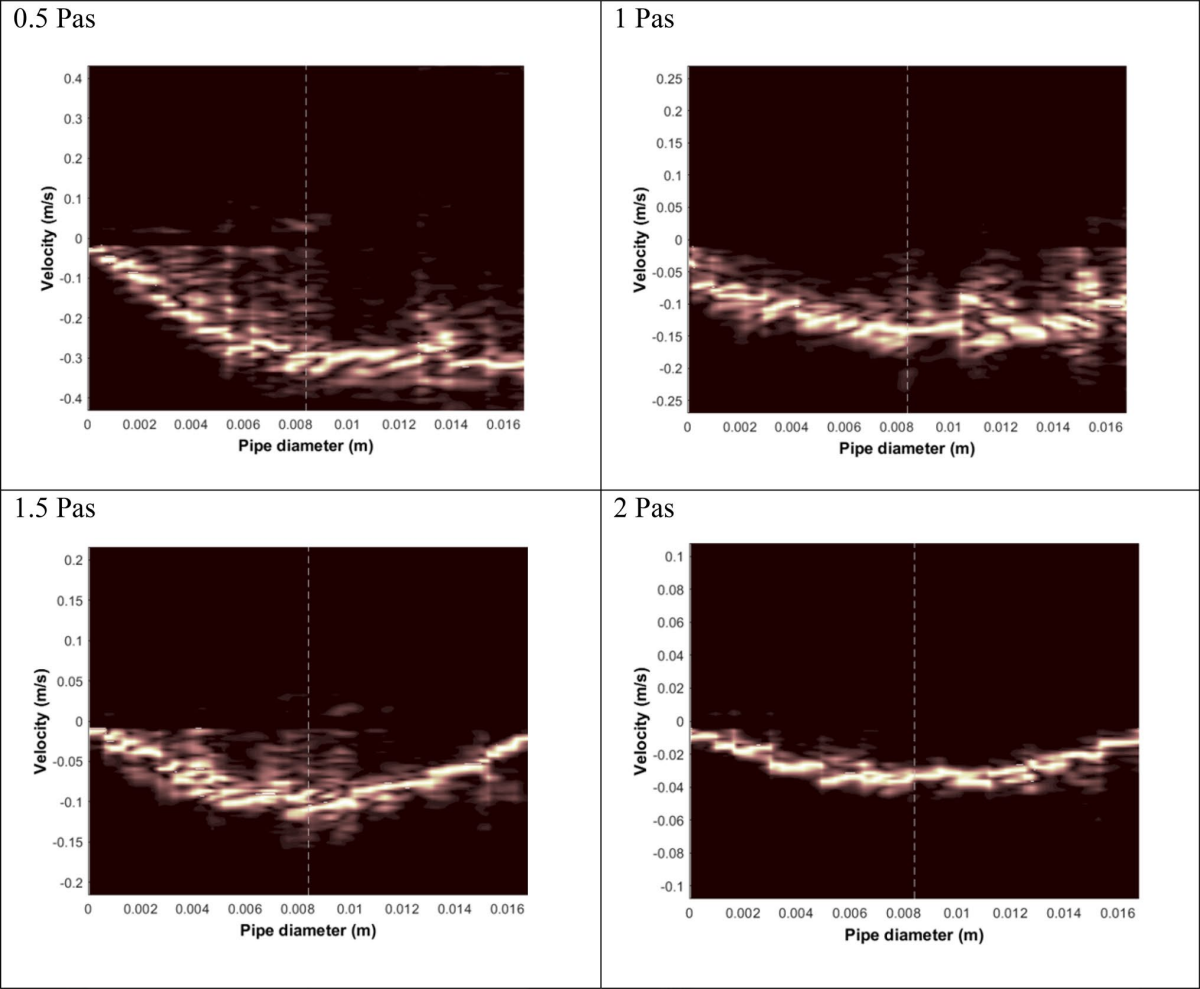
Velocity profiles acquired with ultrasound velocimetry using the Nutilis thickener at viscosities of 0.5, 1.0, 1.5, and 2.0 Pa s at a shear rate of 50 s^−1^
